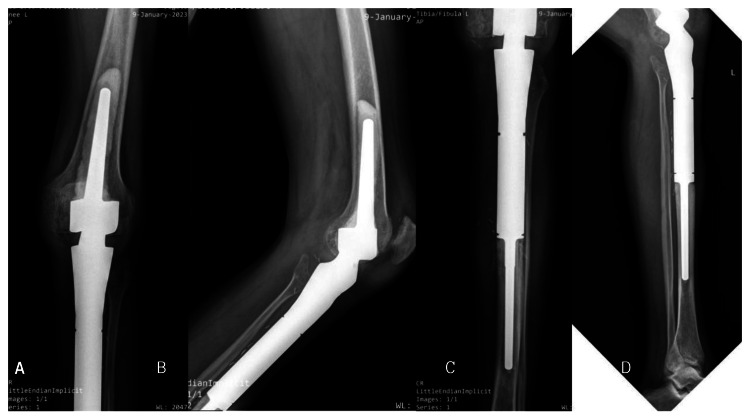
Postoperative radiograph of the left knee with a megaprosthesis in-situ A: Anteroposterior view of the knee, B: Lateral view of the knee, C: Anteroposterior view of the leg, D: Lateral view of the leg
